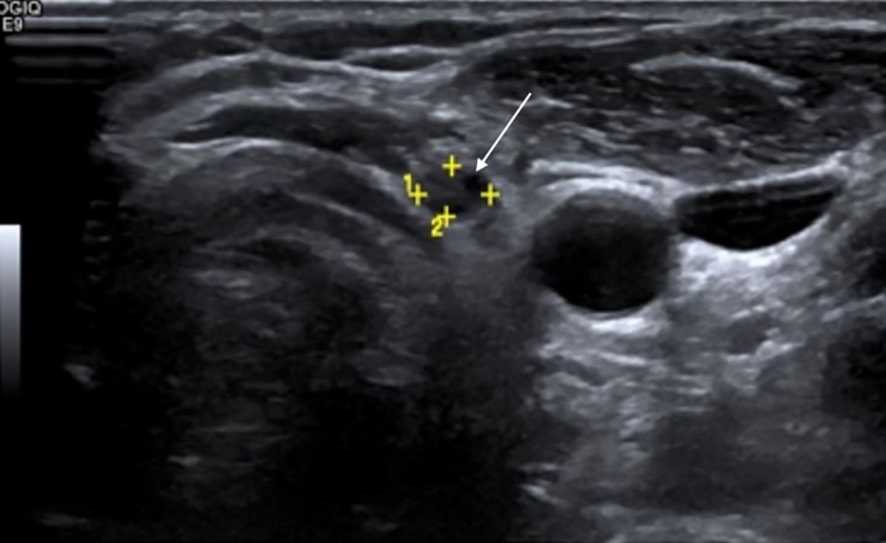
Surveillance greyscale ultrasound of the left thyroid bed in a 28-year-old female with differentiated thyroid carcinoma (DTC) post-total thyroidectomy shows a suspicious hypoechoic nodule with irregular margins (white solid arrow)—features suspicious for recurrence. Serum thyroglobulin (Tg) level was 0.9 ng/mL. Fine-needle aspiration cytology (FNAC) revealed clusters of atypical follicular cells, confirming recurrent disease.
